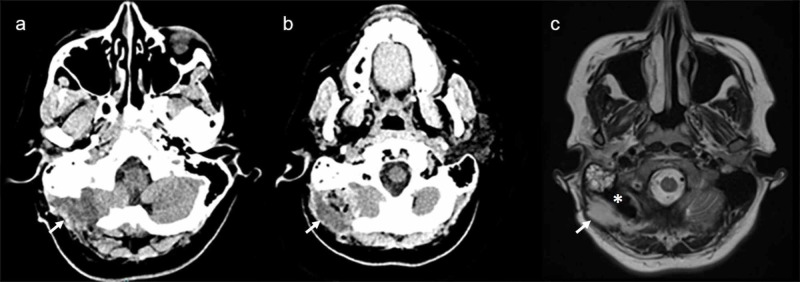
Brain imaging obtained before surgery (a and b) CT imaging of the mastoid area obtained upon presentation showing a large irregular thick-walled mass in the remnant cavity from the dermoid cyst removal 10 years before. (c) Fluid-attenuated inversion recovery (FLAIR) magnetic resonance imaging (MRI) sequence obtained before the first surgery which confirms the presence of a right occipital collection with central restricted diffusion (sequence not shown) and peripheral enhancement, consistent with an abscess formation (arrow) extending to the epidural space, and remnant cavity from the dermoid cyst removal in the past (star).
